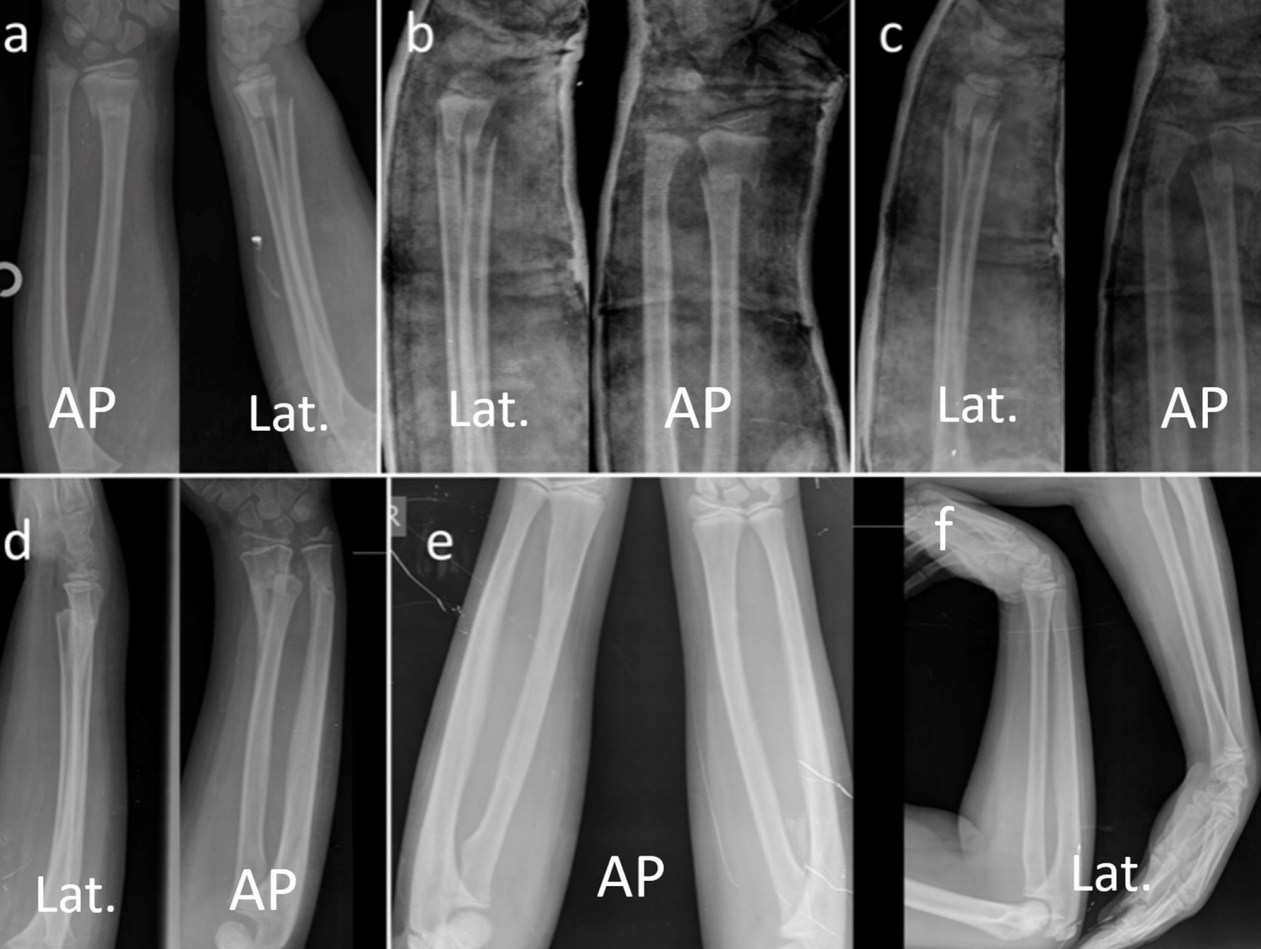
Case #1. Serial radiographic examination of seven-year-old girl with distal radial metaphyseal fracture. (a) Initial radiograph on admission. (b) Immediate closed reduction, the angulations were in acceptable range in both planes. (c) Re-displacement in cast. (d) Radiographs after the cast removal. (e,f) Final comparison radiographs with the un-injured side at 30th month showing full remodeling and normal alignment. AP: Antero-posterior; Lat.: Lateral.
